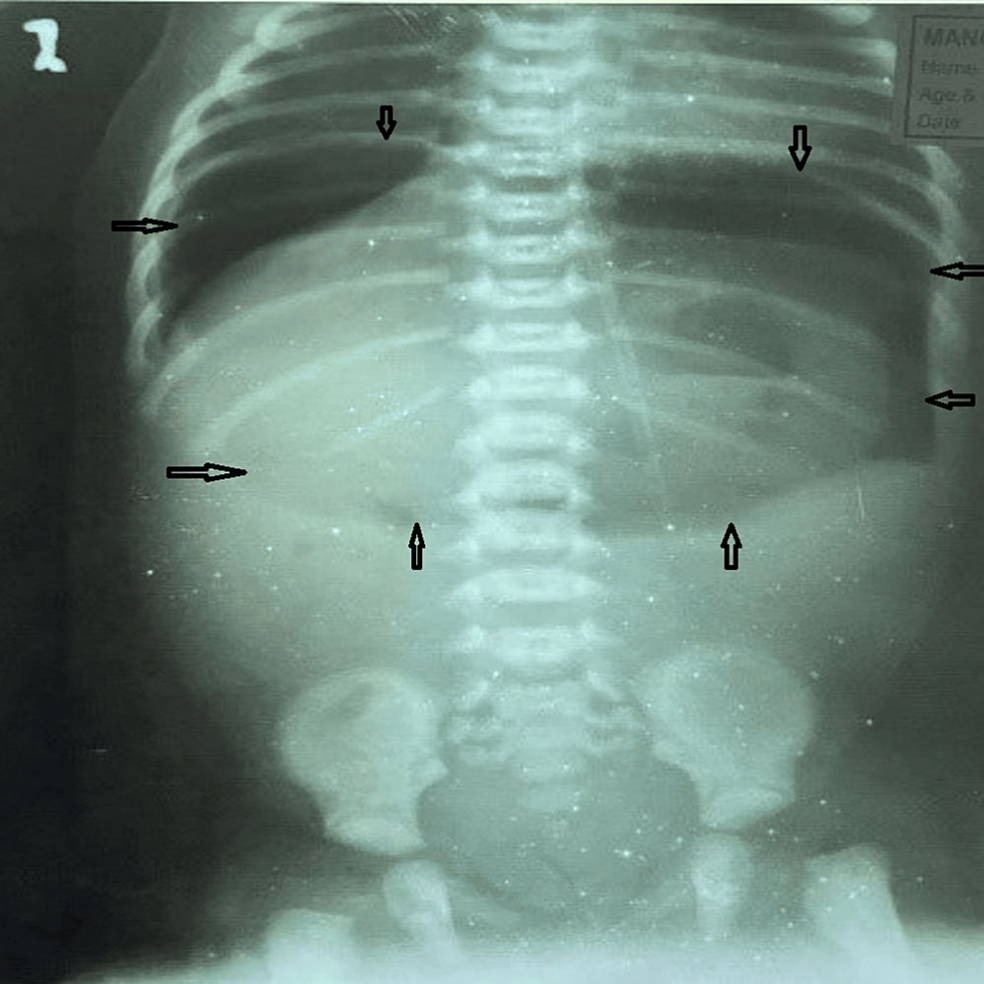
Erect abdominal X-ray of case 4 Erect X-ray in case 4 revealed the “continuous diaphragm sign” and “football sign.” Arrows outline the “football” silhouette.
